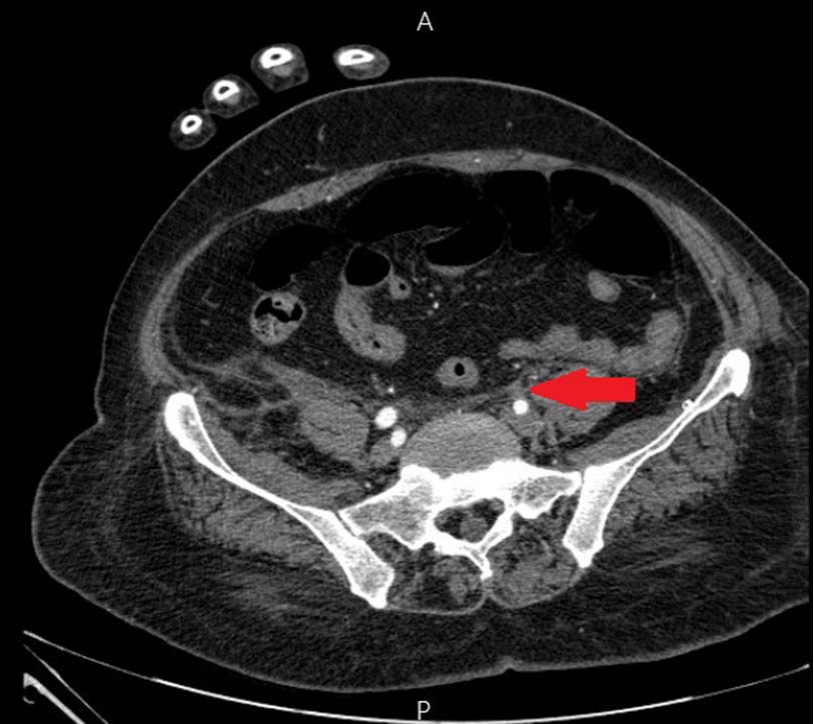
Axial pelvic computed tomography-angiogram showing occlusion in the left external iliac artery (the red arrow).
